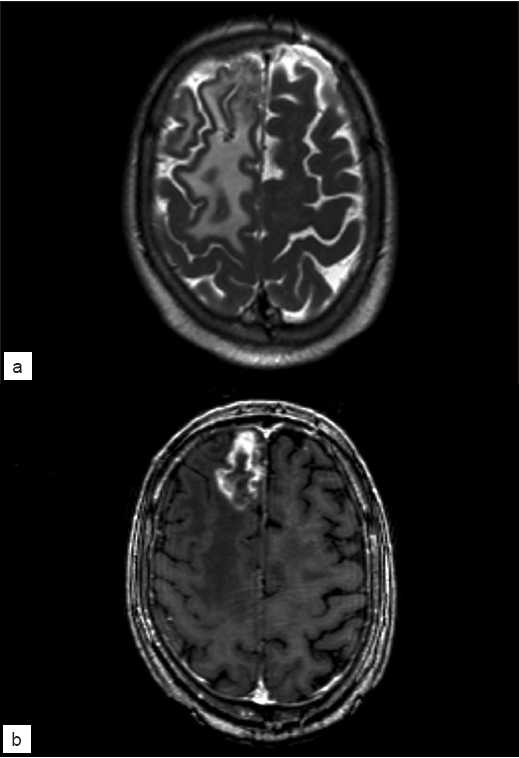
T2 (a) and T1 post-contrast (b) MRI obtained at the time of presentation, demonstrating edema throughout the right fronto-parietal region with an enhancing, necrotic lesion within the anterior right frontal lobe. These imaging features are typical of radiation necrosis
